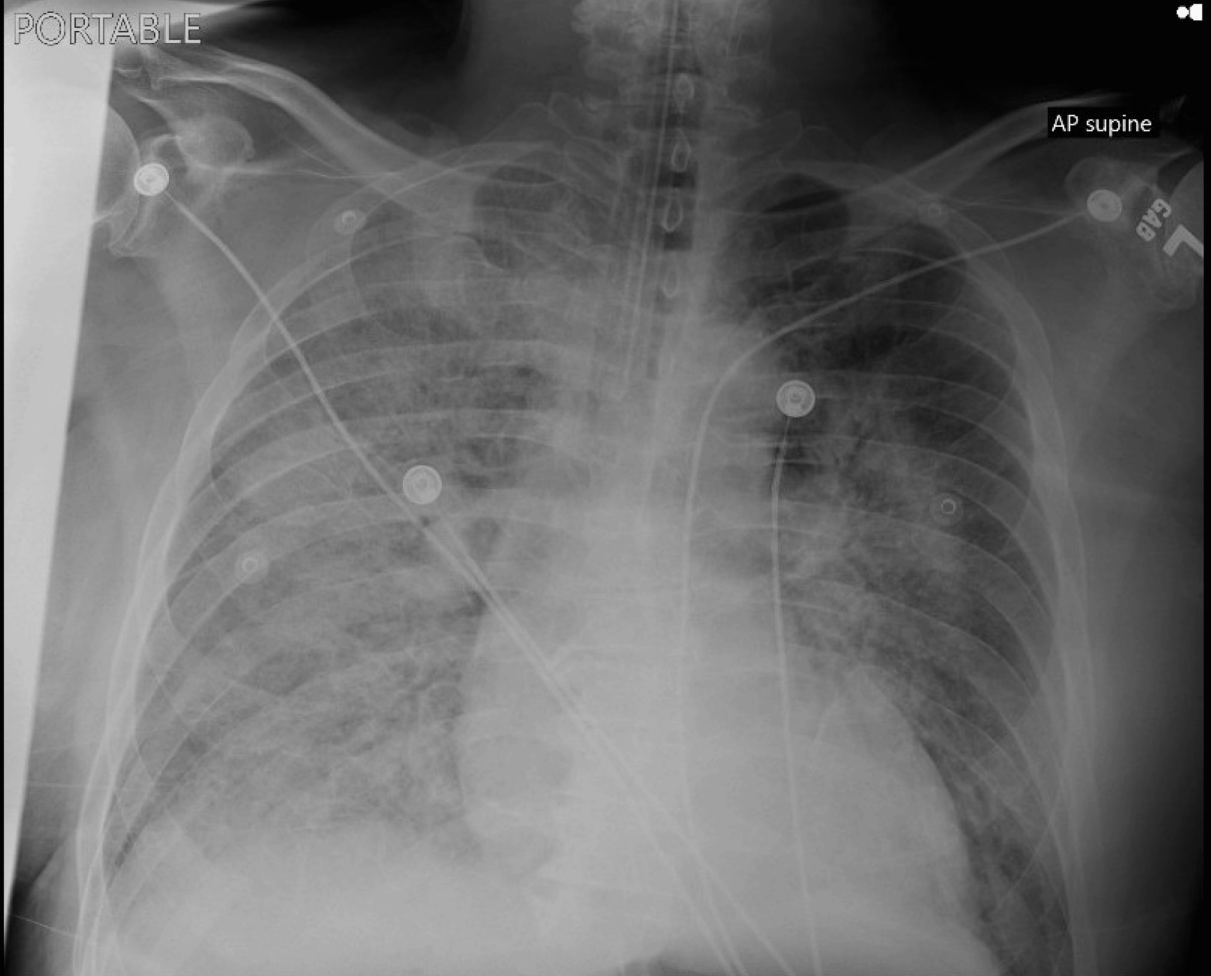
Chest X-ray showing bilateral pulmonary edema, right more than left
